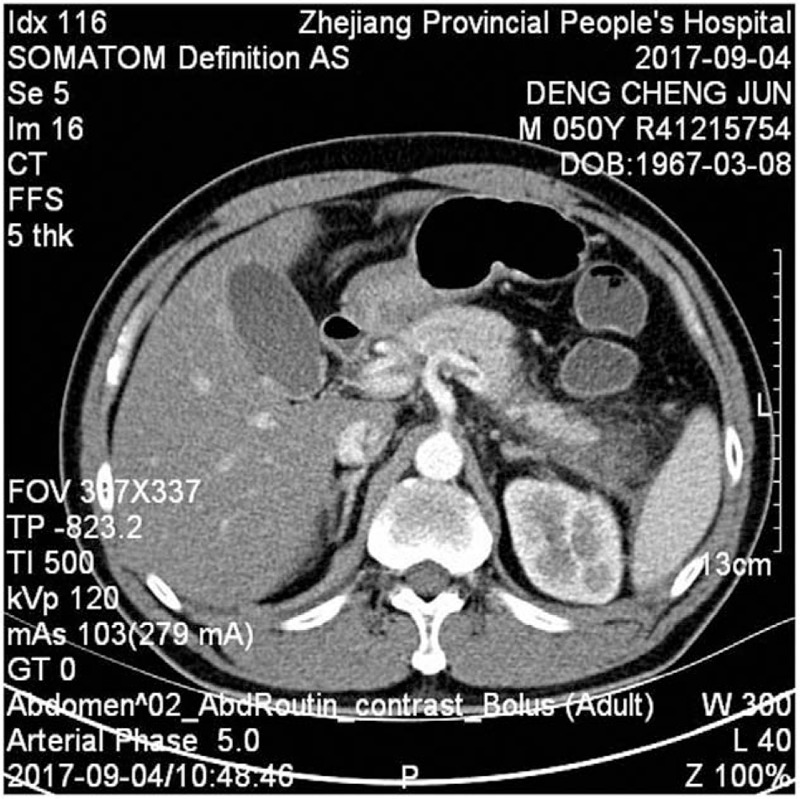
Abdominal computed tomography showing a hypodense lesion in the pancreas surrounded by a moderate amount of peripancreatic fluid.
